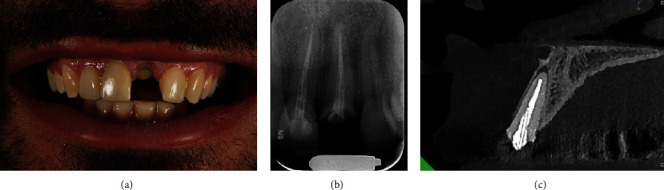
(a) Perioperative clinical examination showing a fractured tooth #9. (b) Perioperative periapical radiograph showing inadequate endodontic treatment with a fractured fiber post in the root canal. (c) Perioperative CBCT assessment. The sagittal slice of tooth #9 confirms a radiolucent area with an intact buccal and palatal plate.
